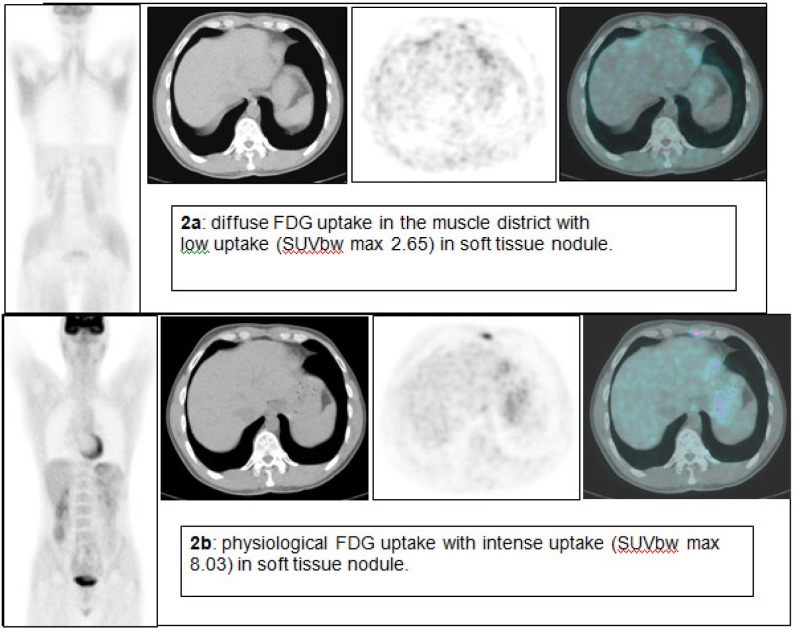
FDG PET/CT of a 38-year-old man with Hodgkin’s disease.
